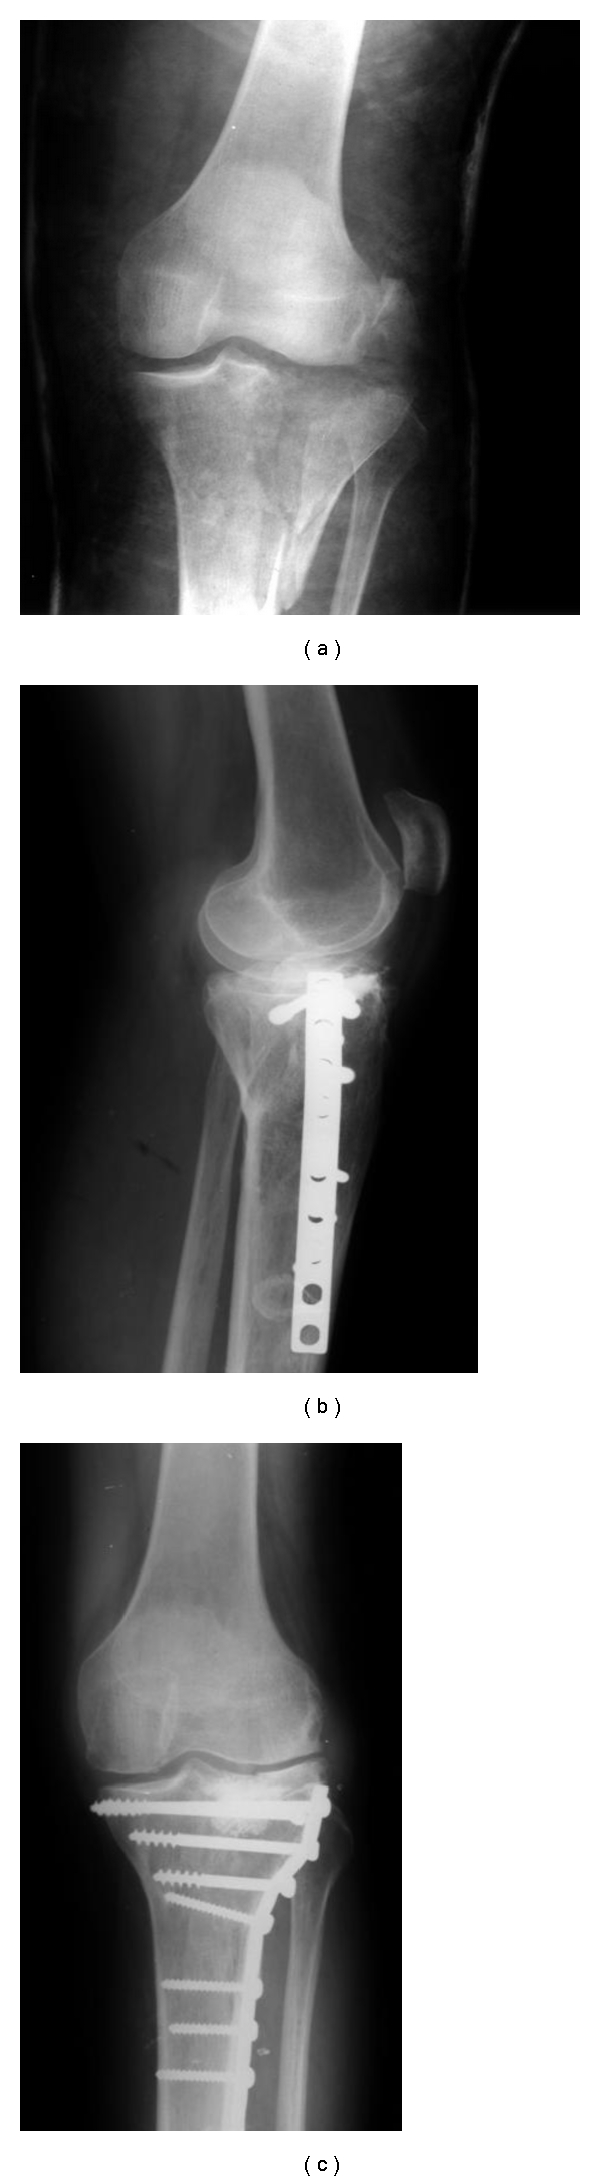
A tibial bone defect reconstructed with BCP. X-Ray image of a tibial fracture (a) with the lateral (b) and anterior view (c) after 2 years of reconstruction using the BCP.
